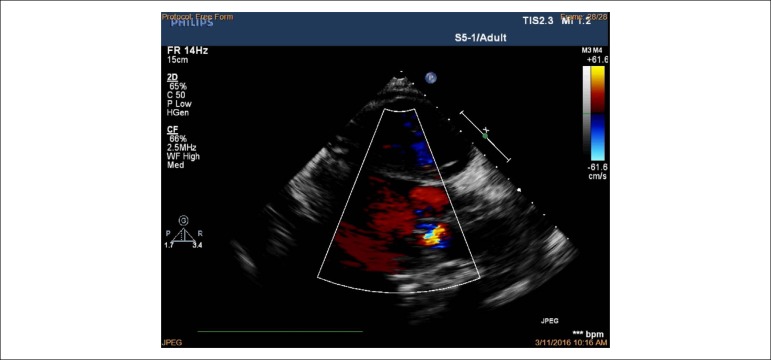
Transthoracic echocardiography from the parasternal long axis view
